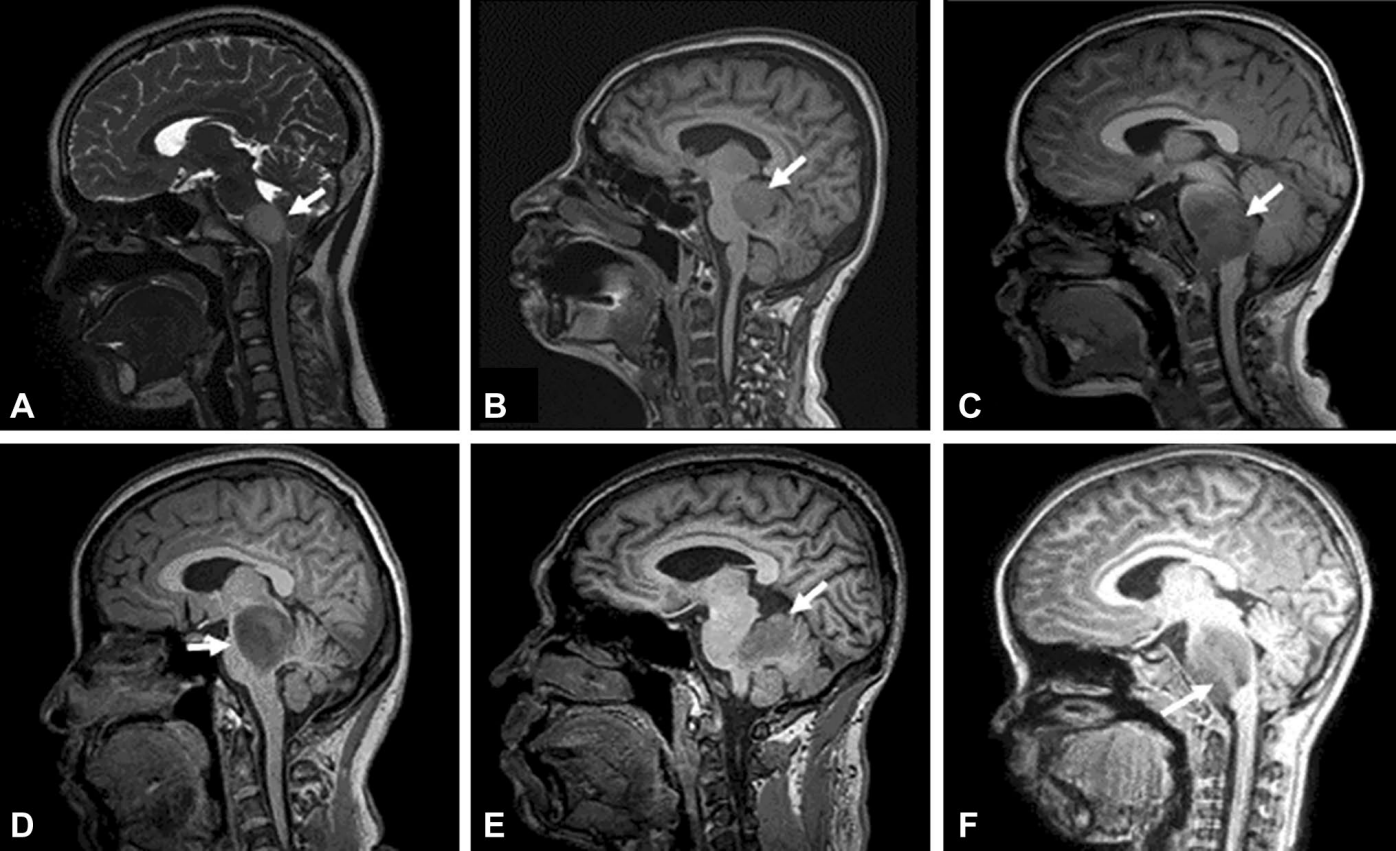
Brain MRI of children with a brainstem tumor. A T2-weighted Sagittal FLAIR MRI image showing a tumor located in the medulla oblongata. B T1-weighted Sagittal FLAIR MRI image showing a tumor located in the midbrain. C and a tumor located in pons D DIPG extending to the midbrain. E DIPG extending to the cerebellum. F DIPG extending to the medulla oblongata. The white arrows point to the tumor body
